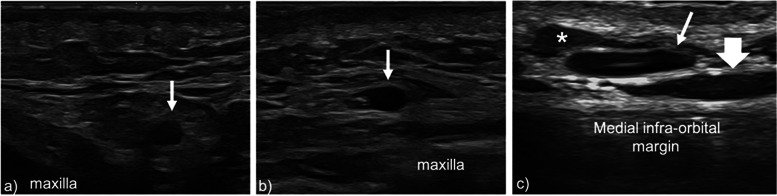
Transverse 22–8 MHz ultrasound images show the facial vein running in the subcutaneous tissue at VP1 (a) and VP2 (b). c Transverse 22–8 MHz US image at VP3 demonstrates the facial vein (white arrow) between the *orbicularis oculi* muscle (asterisk) and the *levator labii superioris* muscle (large arrow). *VP* Venous point
